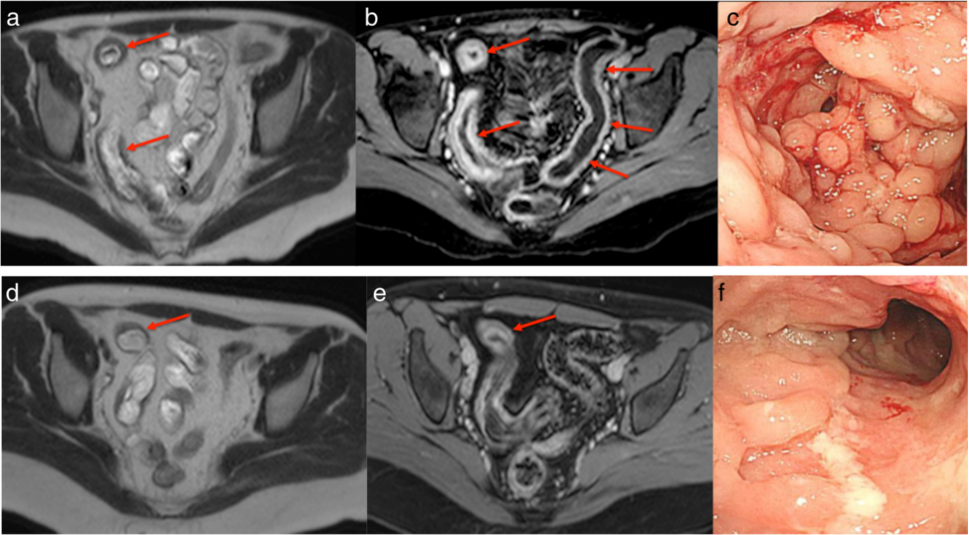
Patient with CD of the distal ileum, left and transverse colon in treatment with IFX. At baseline, MRI (a and b) detected severe inflammatory lesions of the terminal ileum, with marked wall thickening accompanied by oedema, extensive irregularity of the mucosal surface and stratified hyperenhancement after intravenous contrast administration. A moderate wall thickening of the left colon is also present with hyperenhancement after intravenous contrast administration (Overall MaRIA score = 62,7). Baseline endoscopy at the distal ileum revealed the presence of stricturing, cobblestone appearance of the mucosal surface (c; SES-CD = 33). At week 26, the distal ileum continues to present at MRI a moderate wall thickening with mild hyperenhancement after intravenous contrast administration (d and e; Overall MaRIA score = 52,6; Δ MaRIA score = 10,1) Endoscopy of the same segment shows irregular longitudinal ulcers (f; SES-CD = 10)
